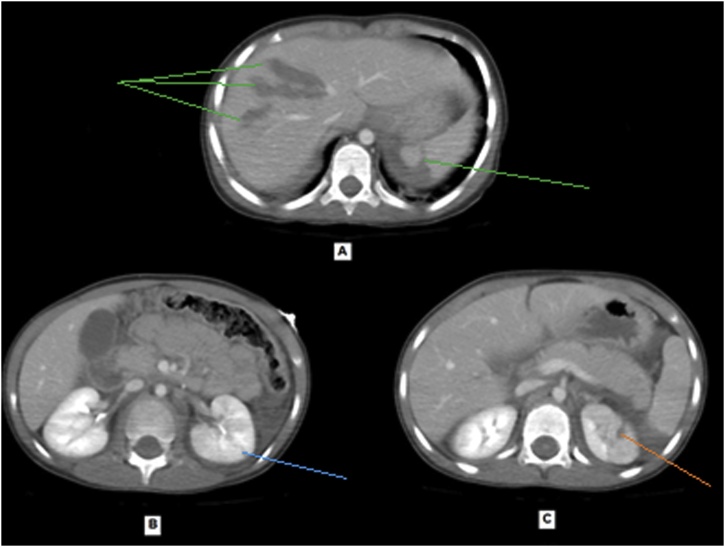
Case 2, CT trauma revealed A, multiple liver lacerations and splenic laceration (green lines). B, left renal laceration at upper zone (blue line) and C, left renal laceration at mid zone (orange line).
